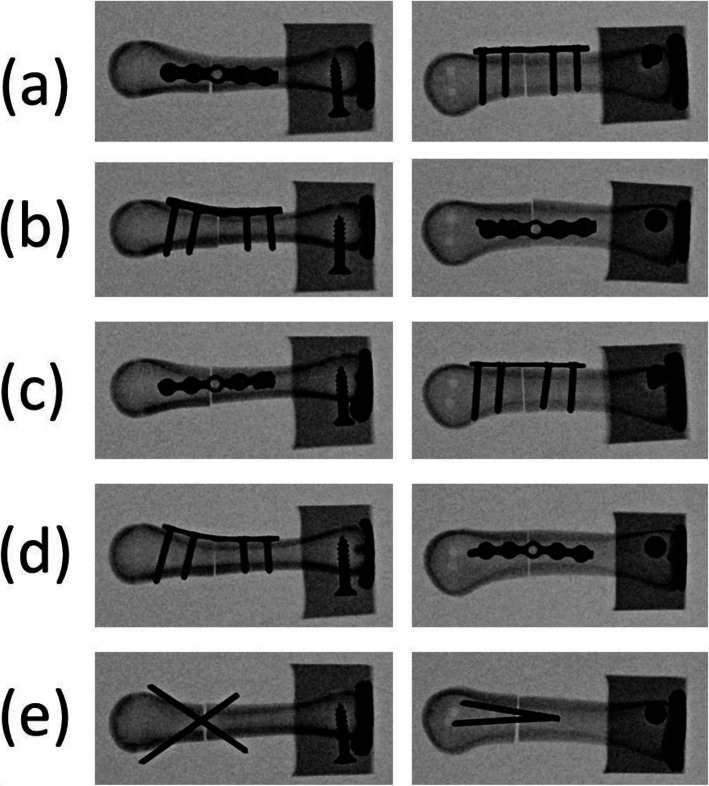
Radiographs of the four fixation types: (a) locking plate with four locking bicortical screws on the dorsal side; (b) locking plate with four locking bicortical screws on the lateral side; (c) regular plate with four regular bicortical screws on the dorsal side; (d) regular plate with four regular bicortical screws on the lateral side; and (e) two K-wires. Left column: anterior–posterior view; right column: lateral view
